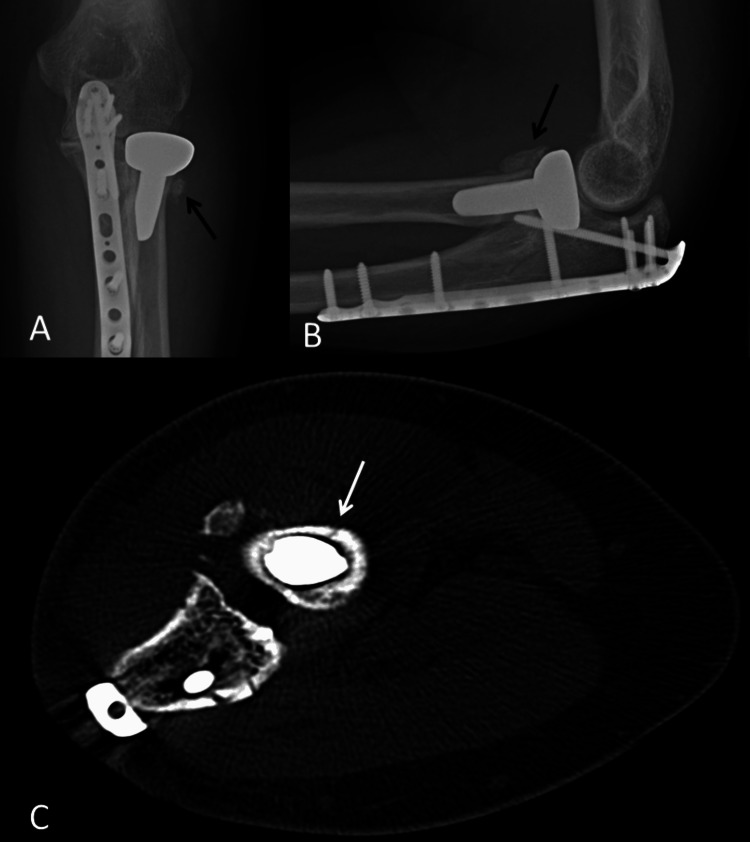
Post-operative radiograph and CT scan. A: Antero-posterior view at four weeks after surgery demonstrating callus formation lateral to the radial neck (black arrow). B: Lateral view at four weeks after surgery demonstrating callus formation (black arrow) and a reduced elbow joint. C: CT scan slice taken eight weeks after surgery, demonstrating an undisplaced fracture line through the radial neck at the level of the radial RHR (white arrow). RHR, radial head replacement
